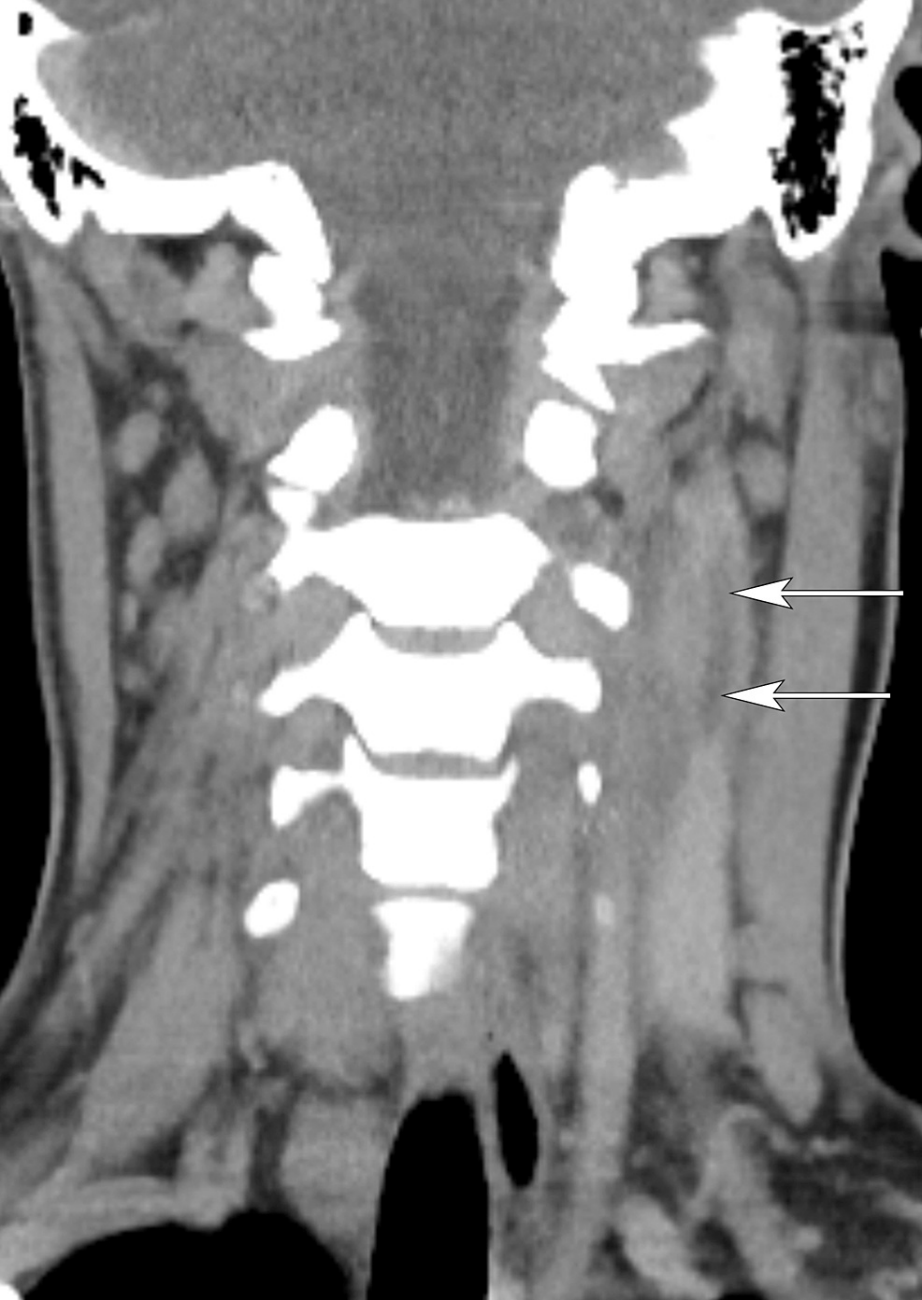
Coronal CT of the neck Coronal postcontrast CT through the left internal jugular vein confirms linear low-density thrombus (arrows).
